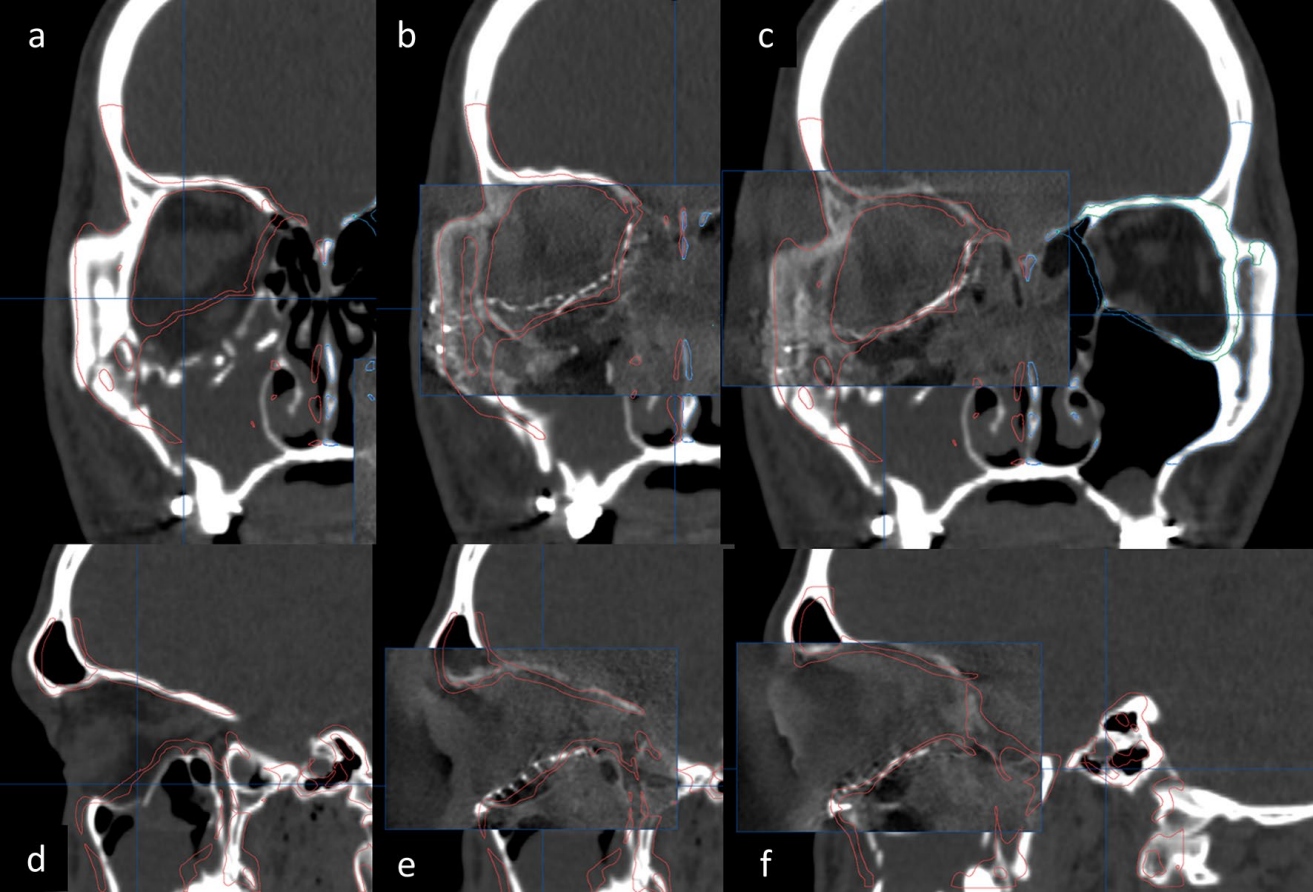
The implant adjustment of orbital reconstruction after intraoperative 3D C-arm computed tomography use. The right orbital floor fracture was shown on the coronal and sagittal view (a,d). After reconstruction, the 3D C-arm CT was applied, and the result revealed that the implant position was not in conformity with the presurgical plan (b,e). The adjustment was made for better reconstruction (c,f).
